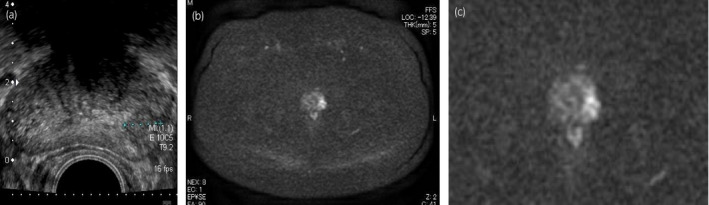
Imaging of the prostate. (a) TRUS of prostate, left peripheral zone suspicious for prostate carcinoma. (b) MRI of prostate, DWI, left peripheral zone suspicious for prostate carcinoma. (c) MRI of prostate, DWI, left peripheral zone suspicious for prostate carcinoma, magnified.
